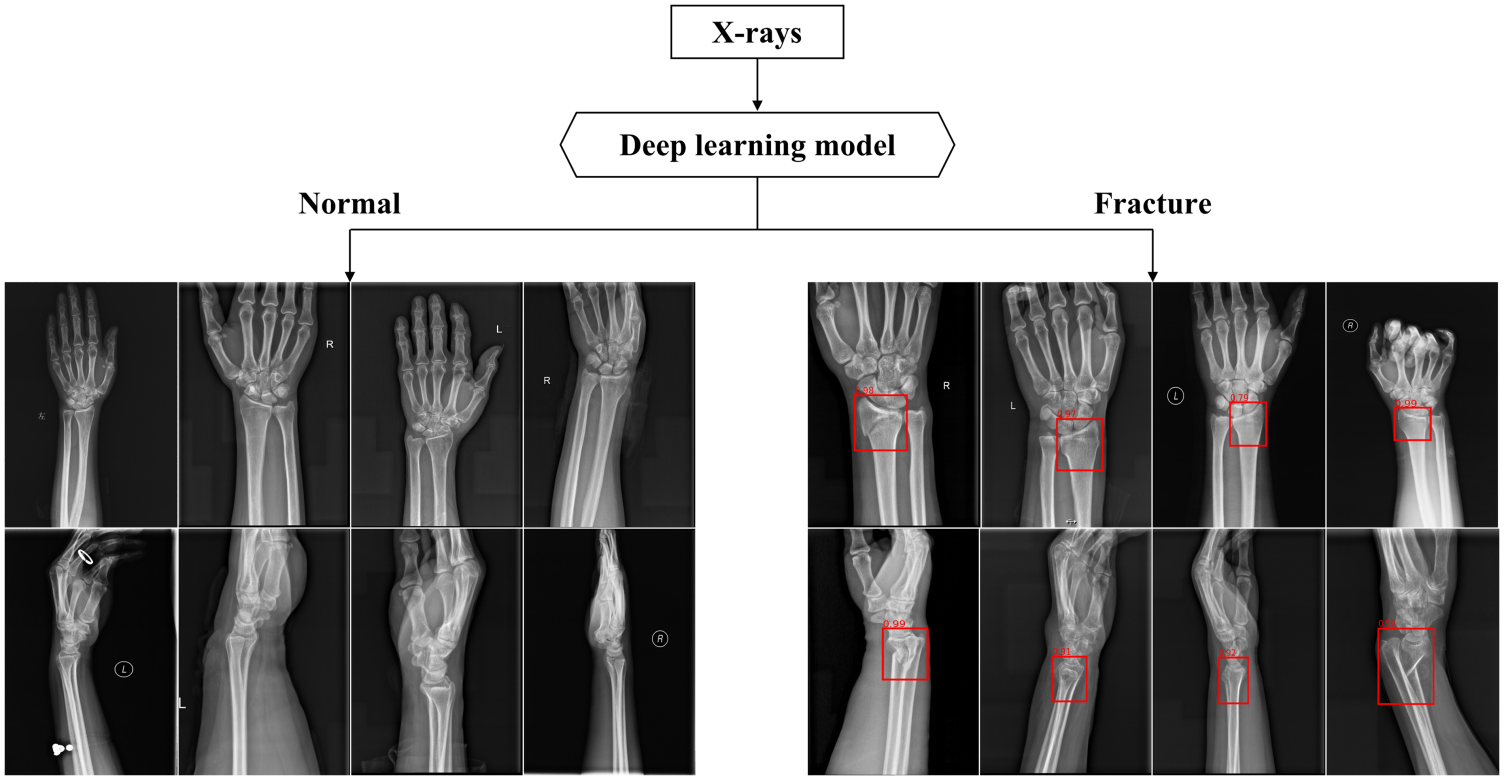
Part of output X-rays dignose results. The above figures show some of the output results in the test dataset. The algorithm used red rectangles to mark suspicious fractures and provided corresponding fracture prediction probability values.
